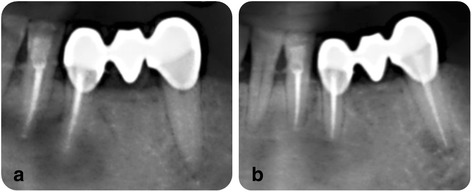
A representative x-ray image of non-healing, a before RCT, b a-year follow-up
